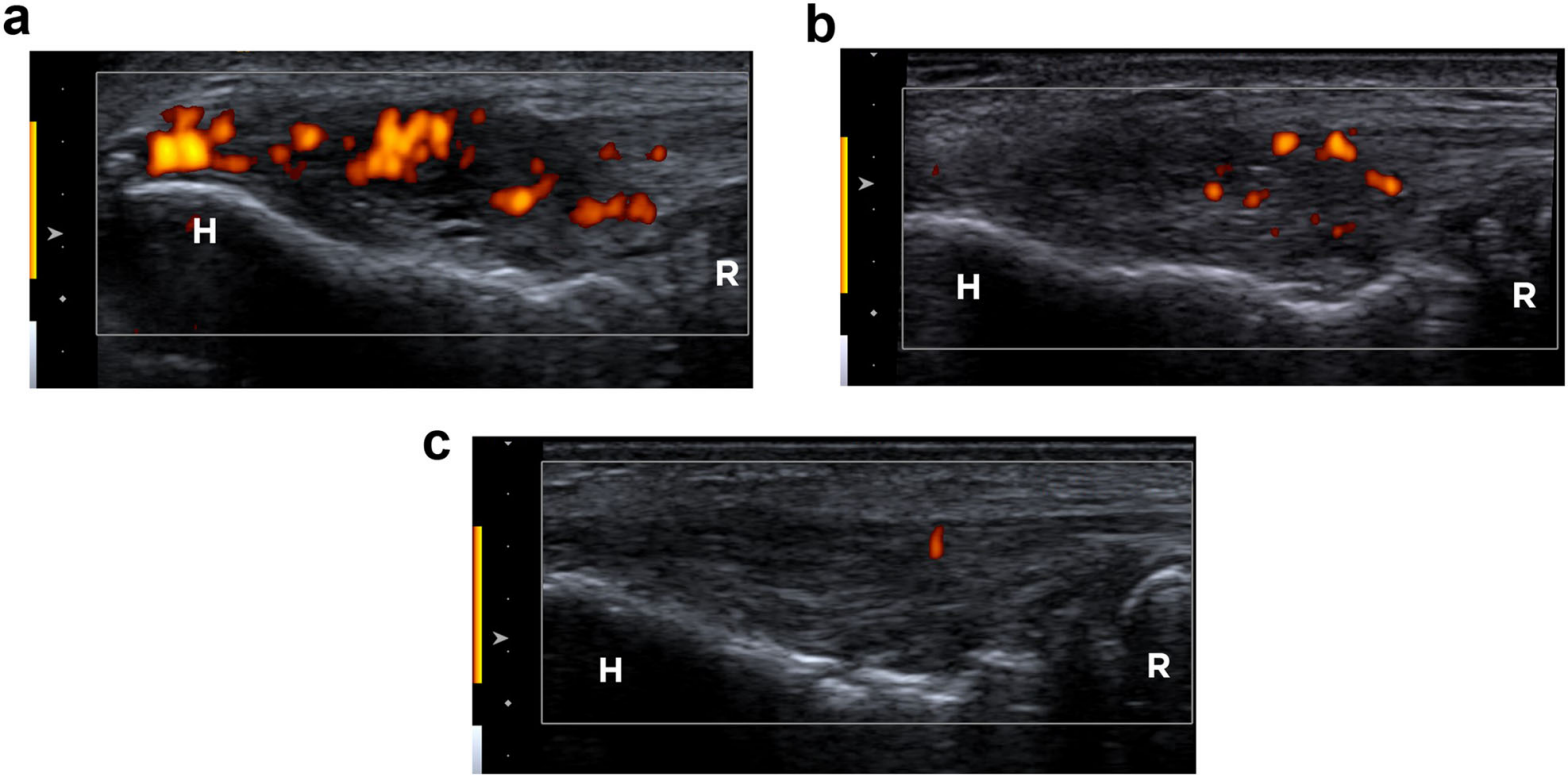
Power Doppler imaging of the right elbow in the same 49-year-old man with chronic lateral tendinopathy. The entire tendon was scanned, capturing the area with the highest neovessel density. a Baseline long-axis US with Power Doppler imaging reveals pixels of Doppler signal covering around 50% of the tendon surface, indicating Grade 2 neovascularity. b Following tendon fenestration treatment, the 6-month follow-up long-axis US displays partial neovessel regression, with a few pixels of Doppler signal indicating Grade 1 neovascularity. c The 12-month follow-up long-axis US exhibits a nearly complete resolution of the Doppler signal (Grade 1 neovascularity)
